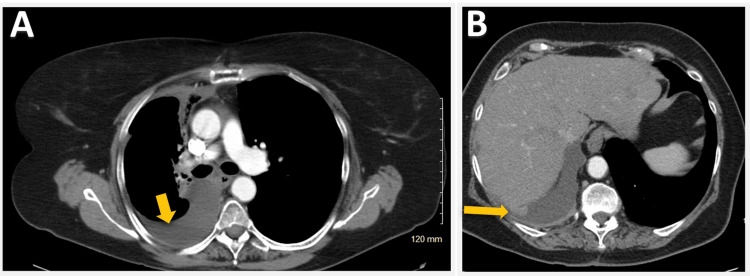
CT scan of the chest showing right lung pleural effusion (yellow arrow in A) and pleural nodularity (yellow arrow in B).
